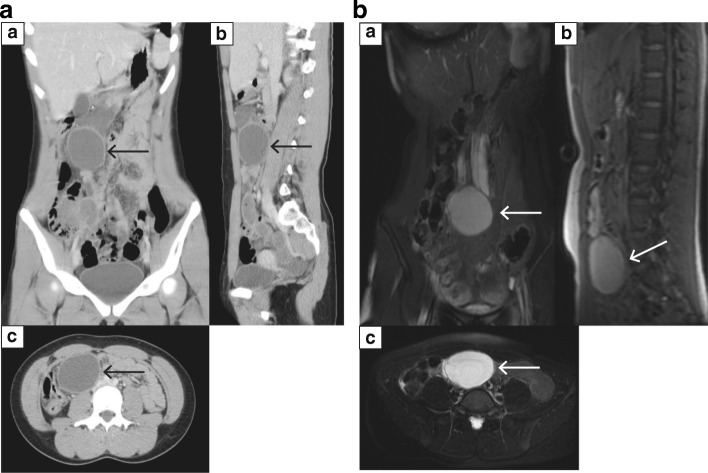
Imaging studies. a Computed tomography of the abdomen. Coronal (a), sagittal (b), and axial (c) images show a well-defined, rounded mass measuring 10 cm along the greatest dimension (arrows). b T2-weighted fast spin echo magnetic resonance image of the abdomen. Coronal (a), sagittal (b), and axial (c) images show a well-defined, rounded mass measuring 10 cm along the greatest dimension (arrows), indicative of a mesenteric cyst
